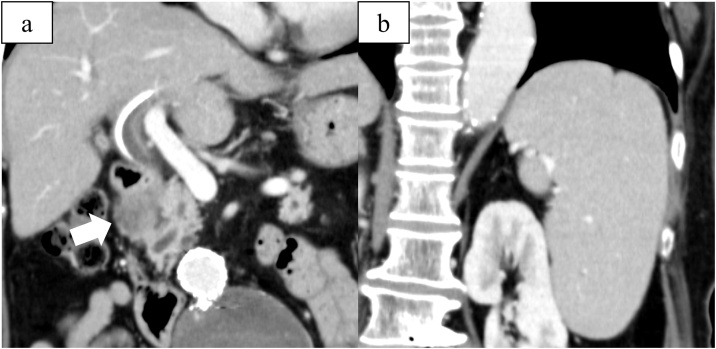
Pretreatment coronal enhanced computed tomography images. a) Hypovascular 25-mm tumour (white arrow) in the pancreatic groove invading the duodenum and lower bile duct. b) Splenomegaly with a maximum splenic diameter of 15 cm, which suggests portal hypertension.
